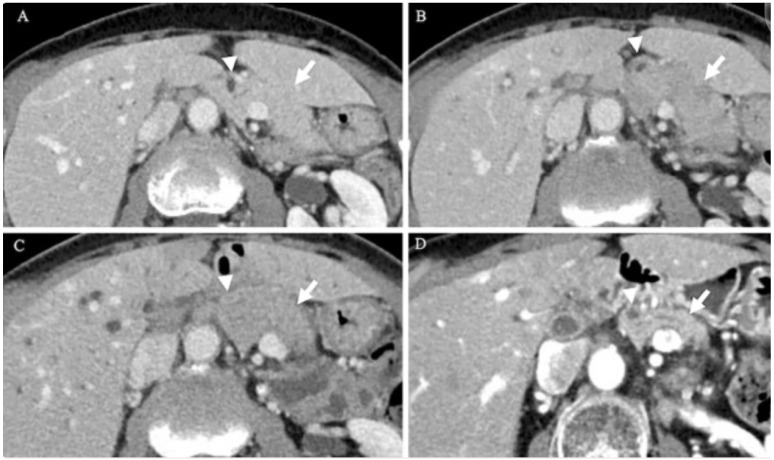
CT scan findings of the pancreas in AIP-3 demonstrated the following changes: (A) Prior to ICI treatment, both the pancreas (arrow) and intrapancreatic bile duct (arrowhead) appeared normal. (B): Following ICI administration, the pancreas showed diffuse enlargement (arrow), and the intrapancreatic bile duct wall appeared thickened (arrowhead). (C) Diffuse enlargement of the pancreas with a capsule-like rim (arrow) and narrowing of the intrapancreatic bile duct (arrowhead) were found. (D): The enlargement of the pancreas (arrow) and narrowing of the intrapancreatic bile duct (arrowhead) improved after the administration of steroids [54].
